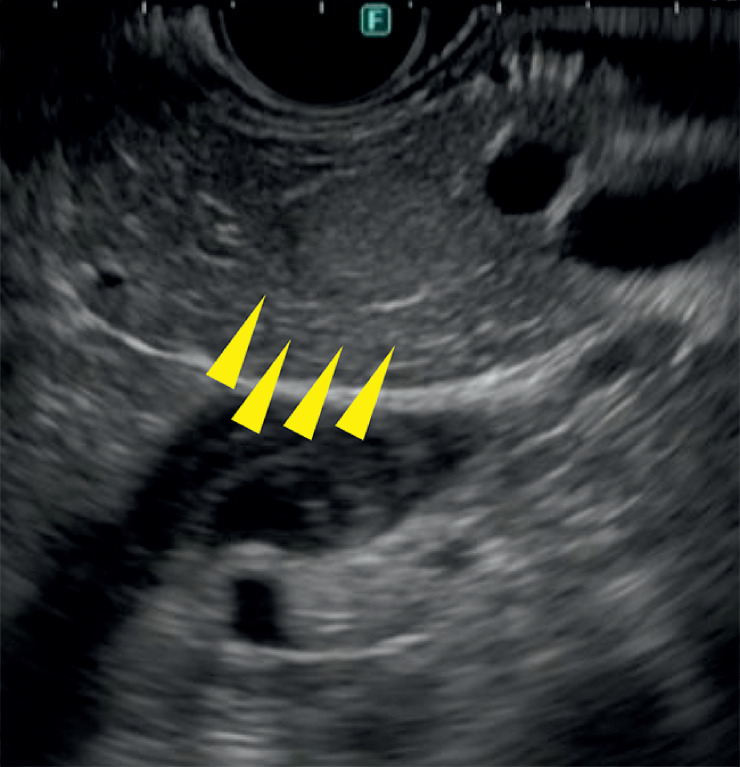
B-mode endoscopic ultrasound showed a 9-mm pale tumor (arrows) with slightly higher echogenicity than the surrounding area.
